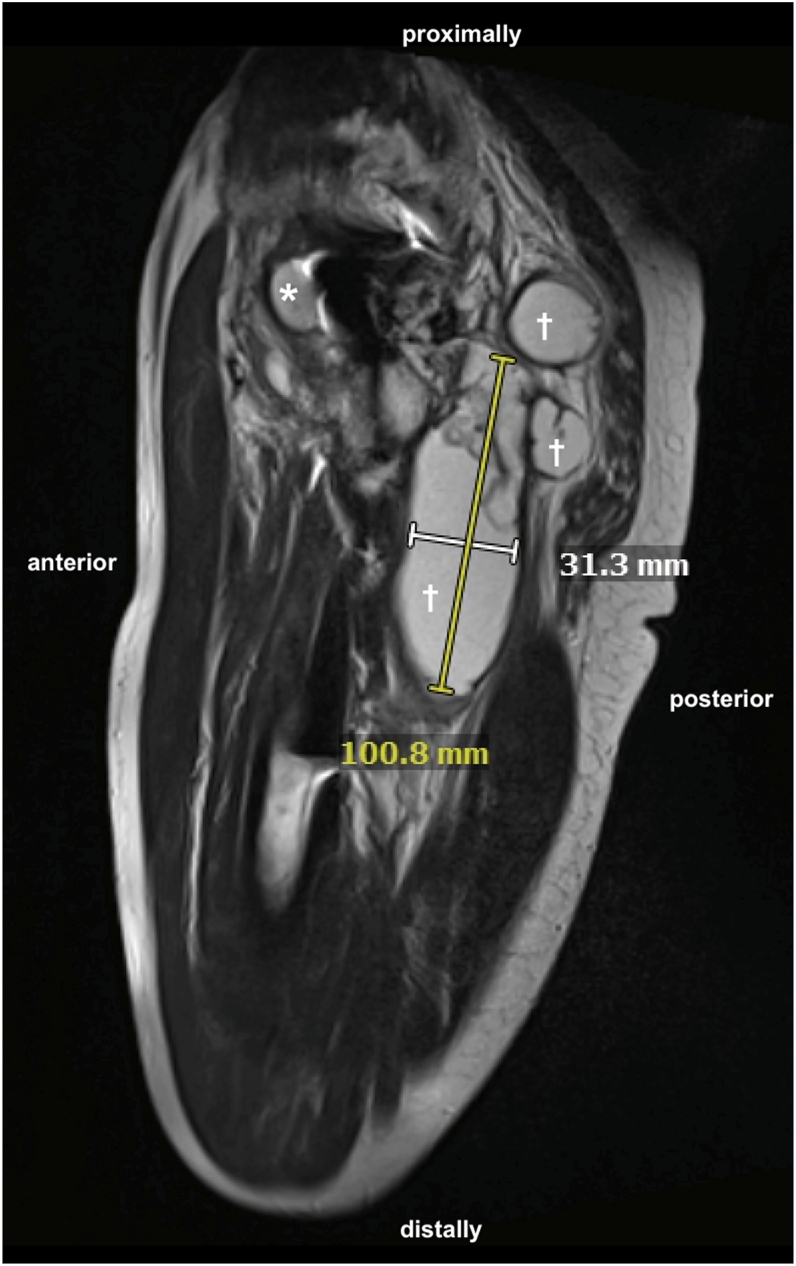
Sagittal view of an MRI with T2 weighting and TSE sequencing of the right proximal thigh in December 2023, revealing a hyperintense multicystic, partly communicating mass measuring up to 100 × 31 mm, located posteriorly (cross) and anteriorly (asterisk) to the hip prosthesis. TSE, turbo spin echo.
